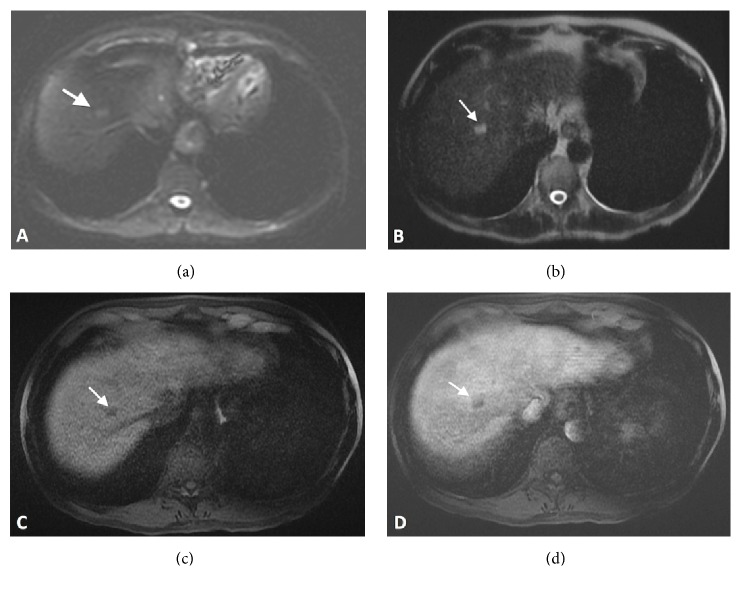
Four axial magnetic resonance images through the liver: (a) diffusion weighted sequence with hyperintense signal in segment 8 lesion showing restricted diffusion, (b) T2-weighted sequence with hyperintense signal within segment 8 lesion, (c) precontrast T1-weighted sequence showing hypointense signal compared to liver parenchyma in segment 8 lesion, and (d) arterial phase postcontrast T1-weighted sequence showing rim enhancement of segment 8 lesion.
